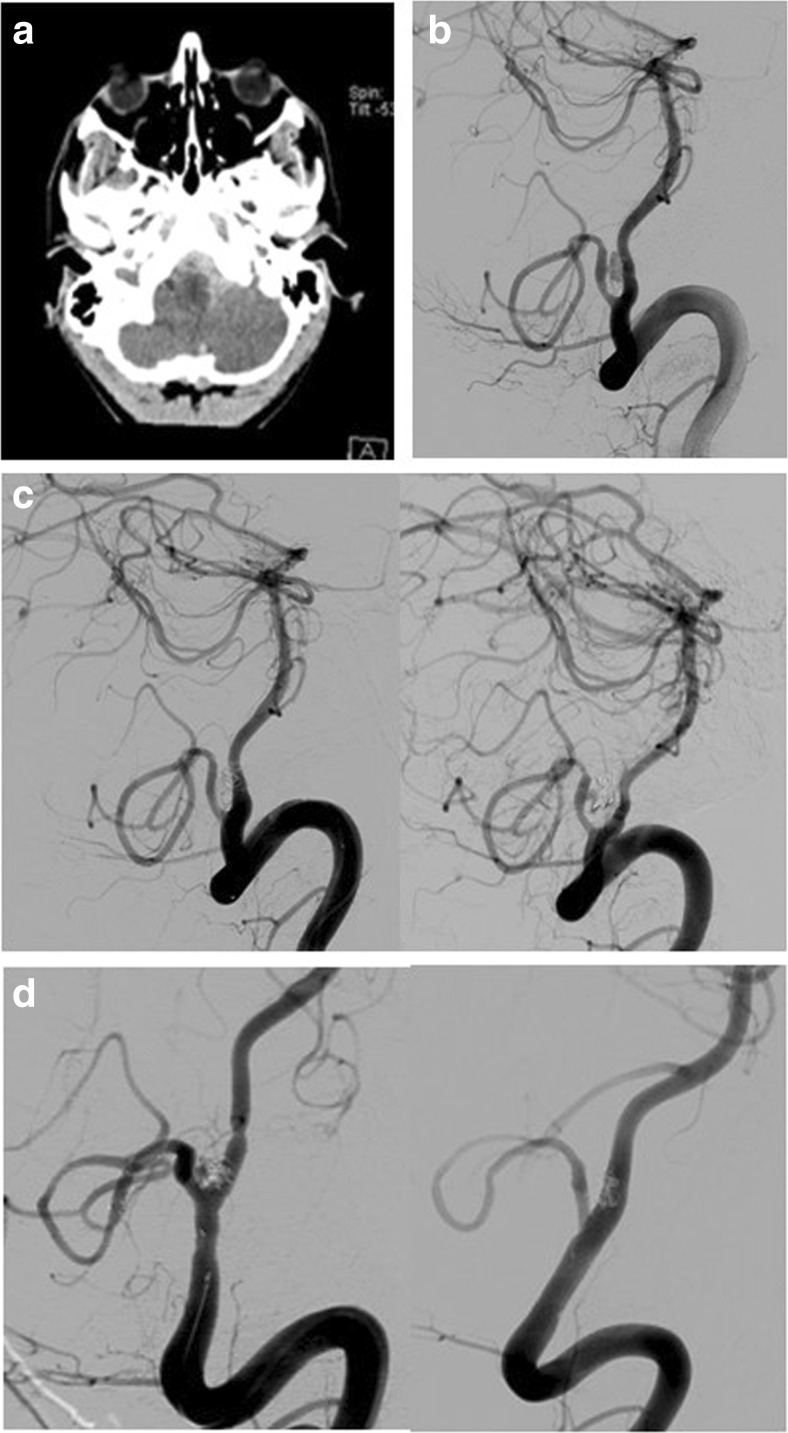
a CT head showing SAH in the posterior fossa cisterns, most prominent around the medulla and hydrocephalus. b DSA demonstrating traumatic PICA dissection and TICA. c A loose coiling of the pseudosac was performed in the first instance (*left*). A DSA was performed 3 days later (*right*) showed an enlarging pseudosac. d Two stents were inserted in the left PICA (*left*). At the end of the procedure there was still some filling of the pseudosac. A follow-up DSA was performed 2 months later (*right*) that demonstrated obliteration of the aneurysm and patency of the PICA
